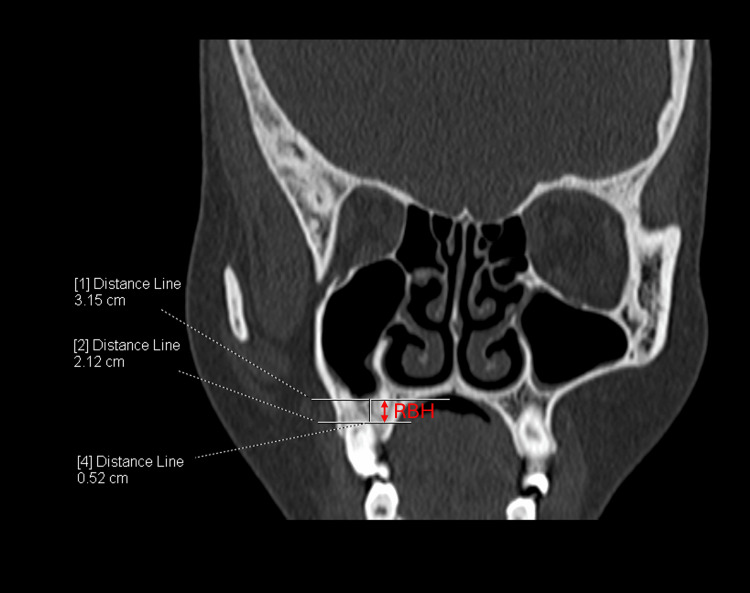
CT section showing the measurement of residual bone height (RBH) in the right maxillary sinus. The RBH is measured as the vertical distance from the alveolar ridge crest to the lowest point of the sinus floor in the coronal section.
